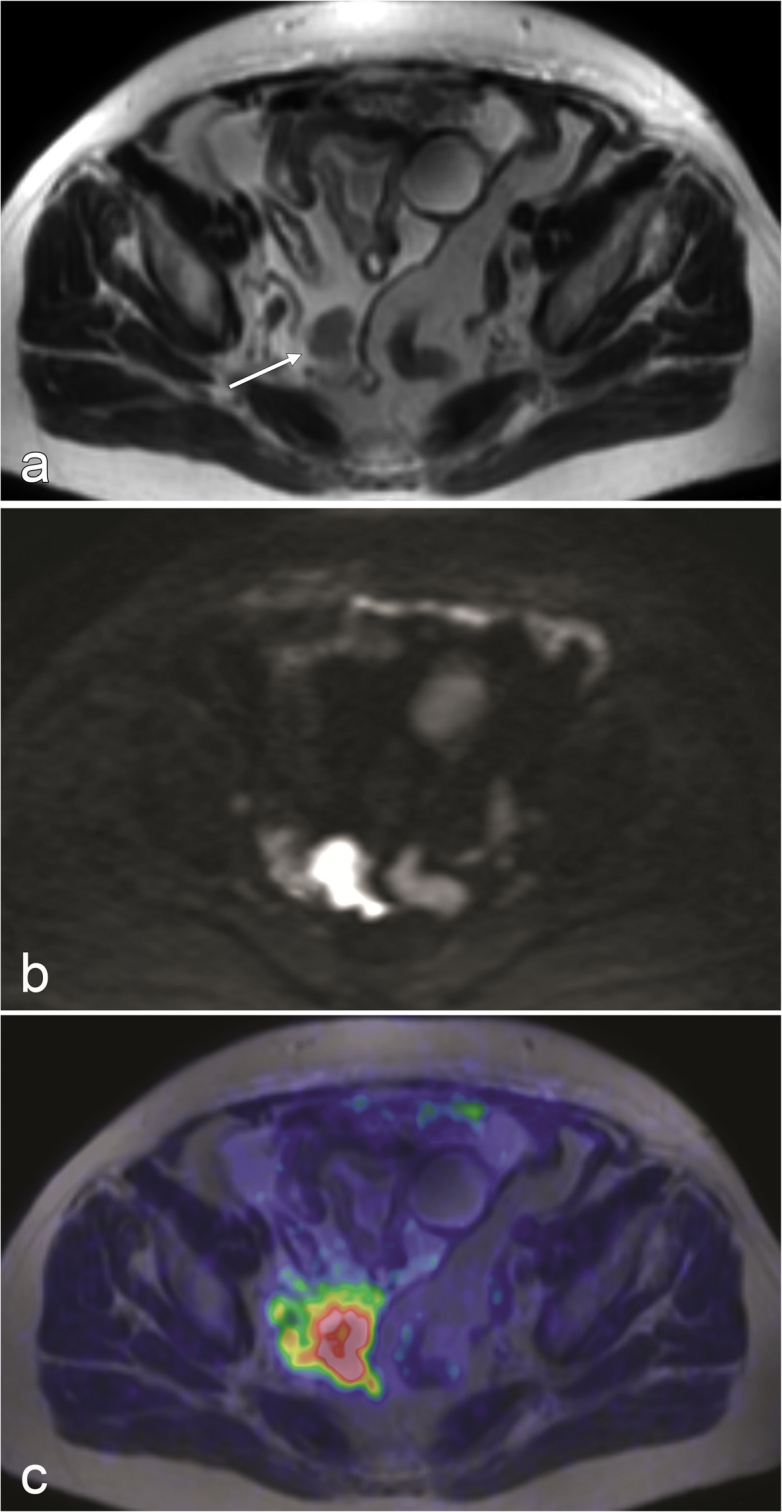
a FSE T2-w image: Ascites helped to correctly localize a peritoneal implant on the surface of pelvic peritoneum (arrow) by displacing adjacent bowel loops. The lesion was easily diagnosed either by MRI alone or by PET/MRI because of clear restriction of diffusion in DWI (b) and marked FDG uptake (c)
